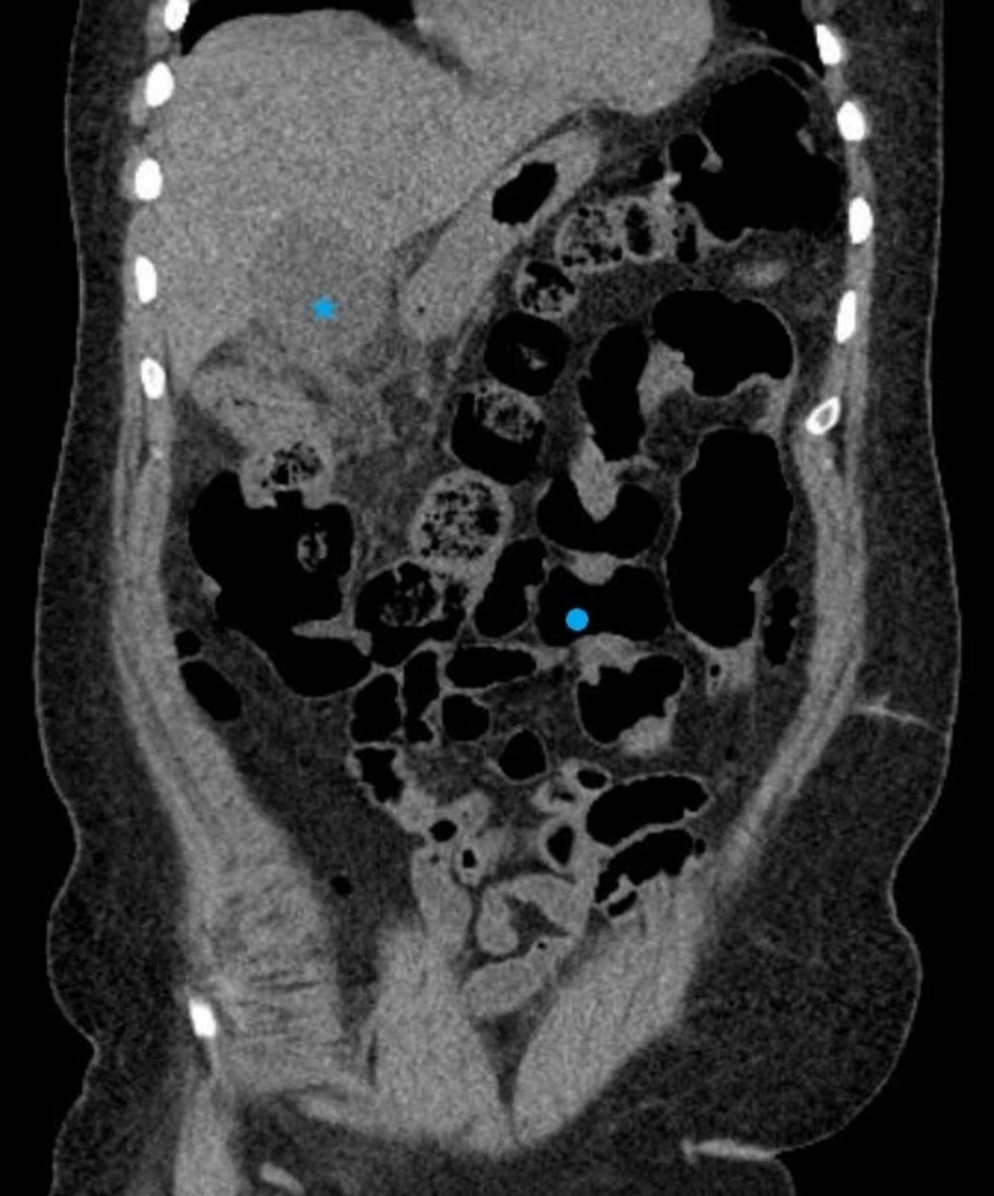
CT scan of the abdomen demonstrating a distended gallbladder (star) and no signs of large bowel perforation (circle)
